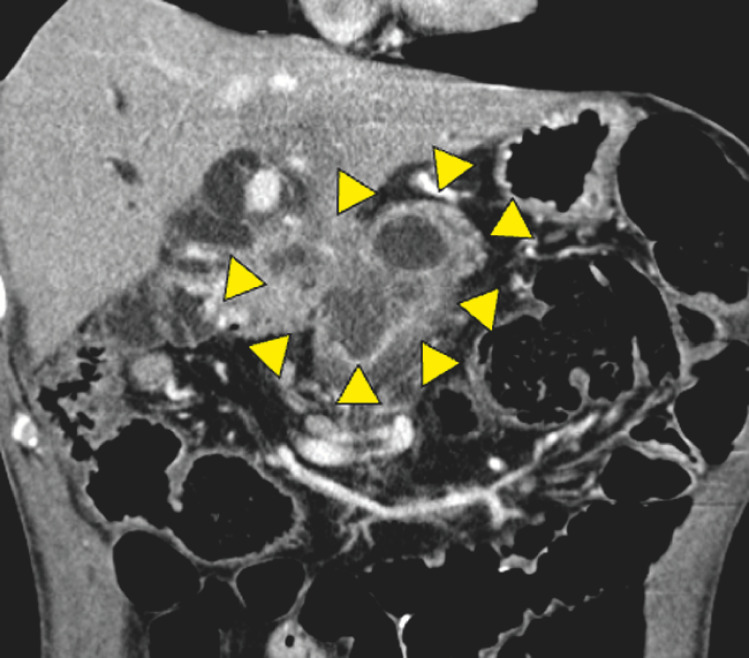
Malignant hilar biliary obstruction by recurrence of cholangiocarcinoma as visualized by computed tomography examination.
